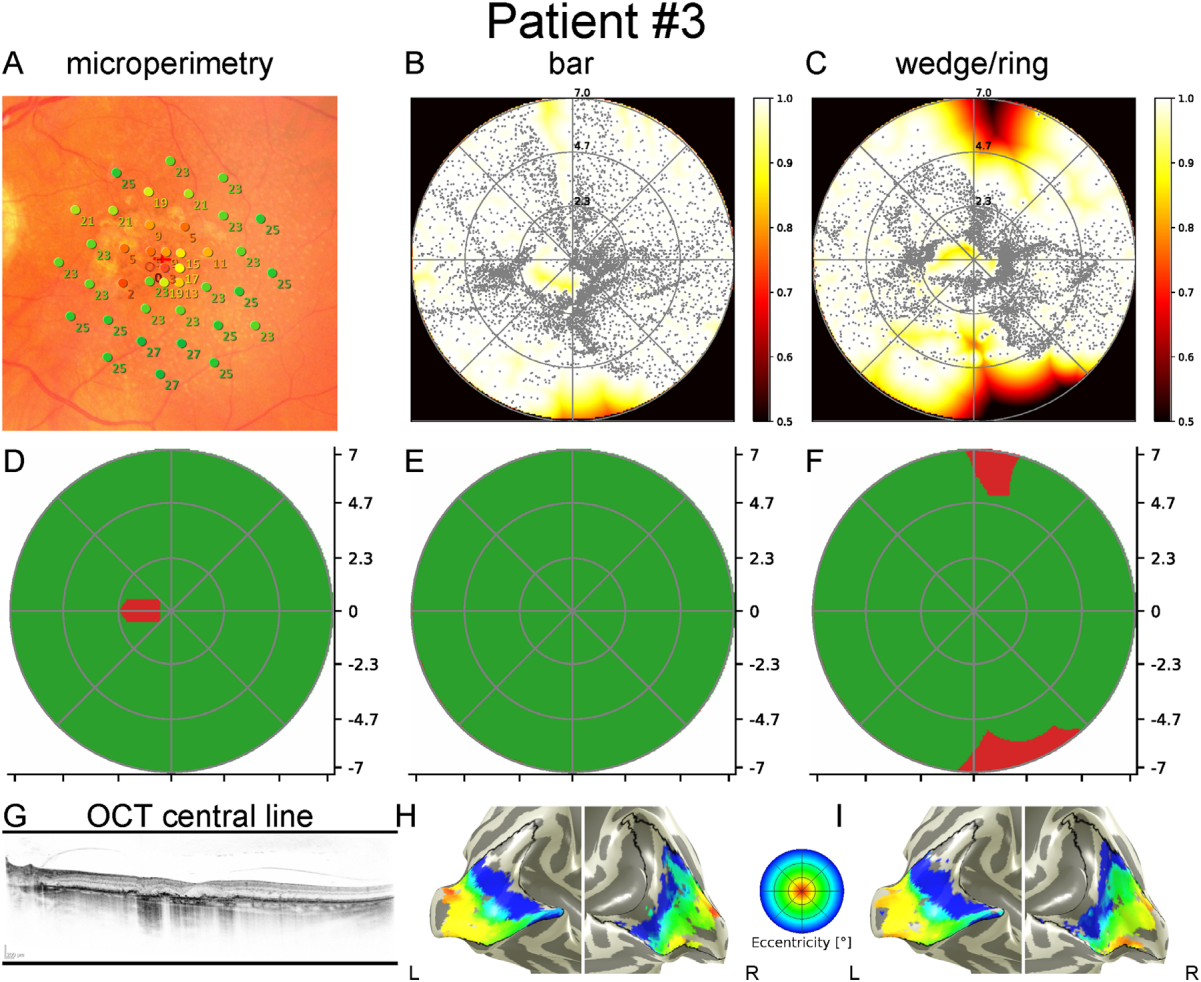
Results of the left eye of patient 3 with geographic atrophy in the superonasal macula. *Top row*: MP overlaid on fundus image (A) (point colors *red* to *green* indicate 0 dB to 34 dB MP results); pRF coverage maps calculated from the functional MRI data with bar (B) and wedge/ring stimuli (C); *Middle row*: Binarized MP (D); binarized pRF coverage maps from bar (E) and wedge/ring stimuli (F); *Bottom row*: Central OCT (G); eccentricity maps from bar (H) and wedge/ring stimuli (I) overlaid on the white matter/grey matter surface mesh. Although the paracentral scotoma is clearly delineated in the distribution of pRF centers on visual field maps, it is not visible in the binarized pRF maps. In the pRF map based on wedge/ring stimuli, the central clustering of pRF centers introduces supposed peripheral visual field defects. There is a clear difference in the distribution of pRF centers between stimuli; pRF centers are distributed homogenously throughout the visual field in visual field maps derived from bar stimuli, but in visual field maps derived from wedge/ring stimuli, they are clustered toward the center (bar: <2.5 degrees: 2640 [40%], >2.5 degrees: 3910 [60%]; wedge/ring: <2.5 degrees 2989 [50%], and >2.5 degrees: 2943 [50%]).
